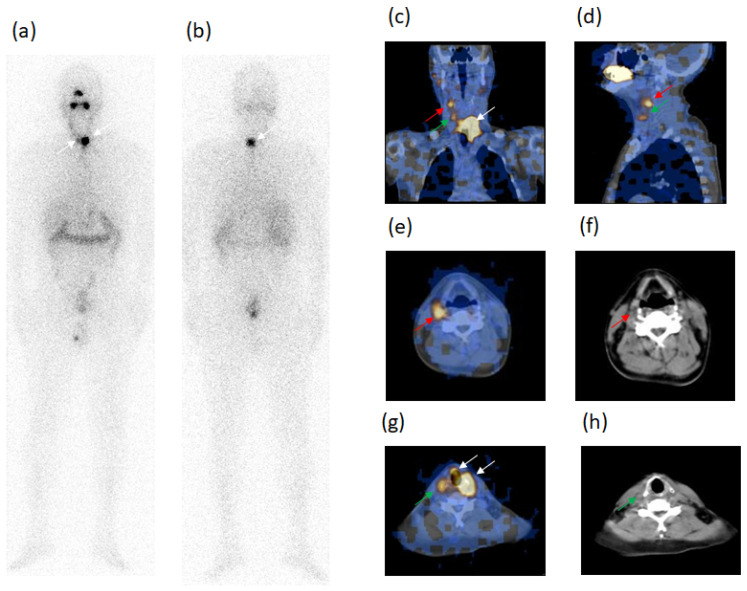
A 42-y-old male patient (case n. 2, Table 3), thyroidectomized for papillary carcinoma, tumor diameter 14mm, at the first radioiodine ablation. ^131^I-WBS after a therapeutic dose, in both anterior (a) and posterior (b) views, showed two radioiodine-avid foci of different dimension and activity in the median region of the neck (white arrows), better evidenced in anterior view and classified as tissue residues in thyroid bed; moreover, a slightly heterogeneous radioiodine uptake was ascertained in right laterocervical region classified as unclear. Fused SPECT/CT images in coronal (c), sagittal (d) and transaxial (e–g) views evidenced the aforementioned two foci (white arrows) in the thyroid bed confirming the classification as residues. SPECT/CT also identified two further circumscribed foci (red and green arrows) in right laterocervical region, whose uptakes were considered unclear by WBS, classifying these as lymph node metastases, as confirmed by CT (f–h). Thyroglobulin levels in hypothyroidism condition: 29.3 ng/mL. AbTg: absent.
